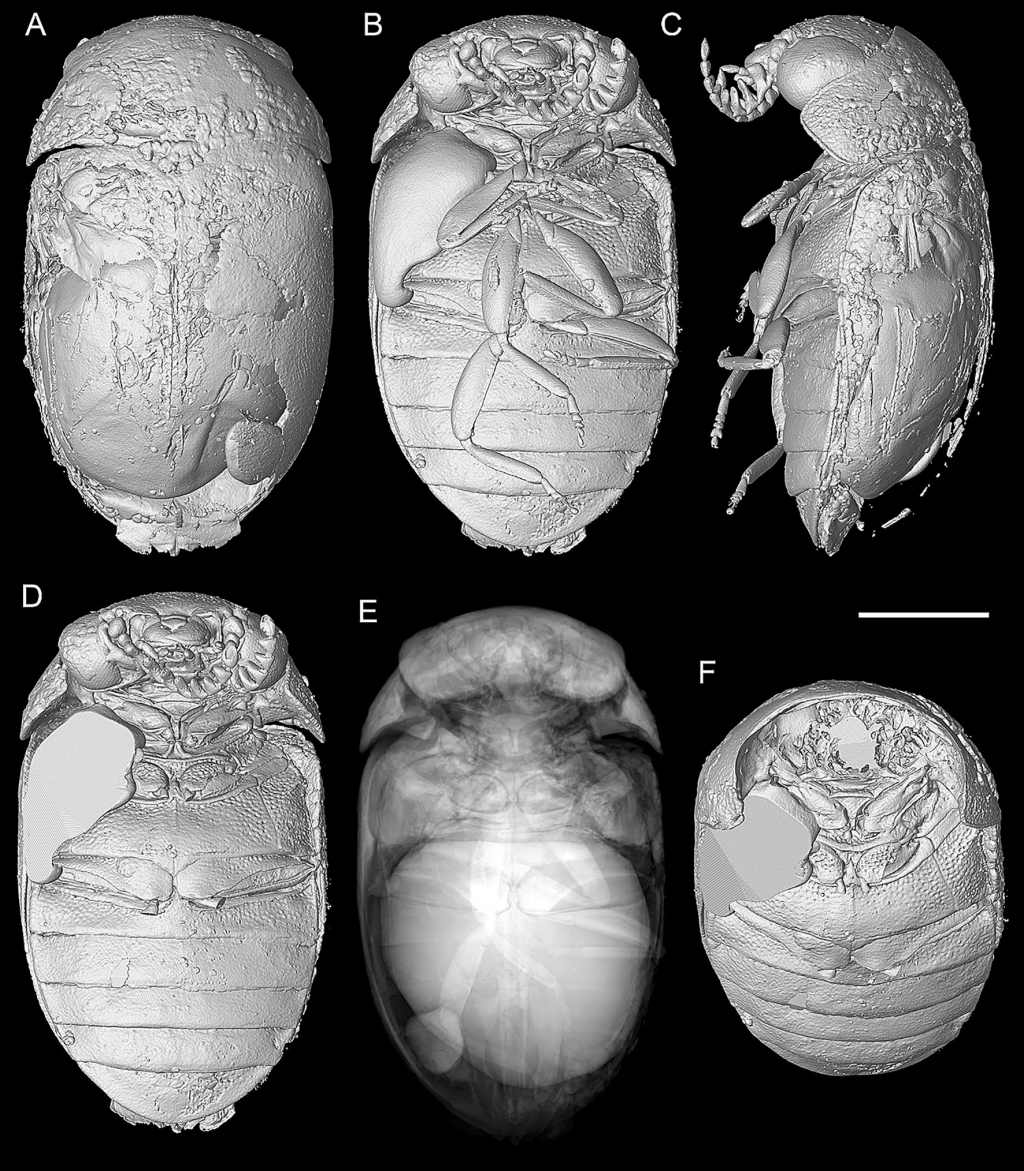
X-ray microtomographic reconstruction of *Varcalium lawrencei*
